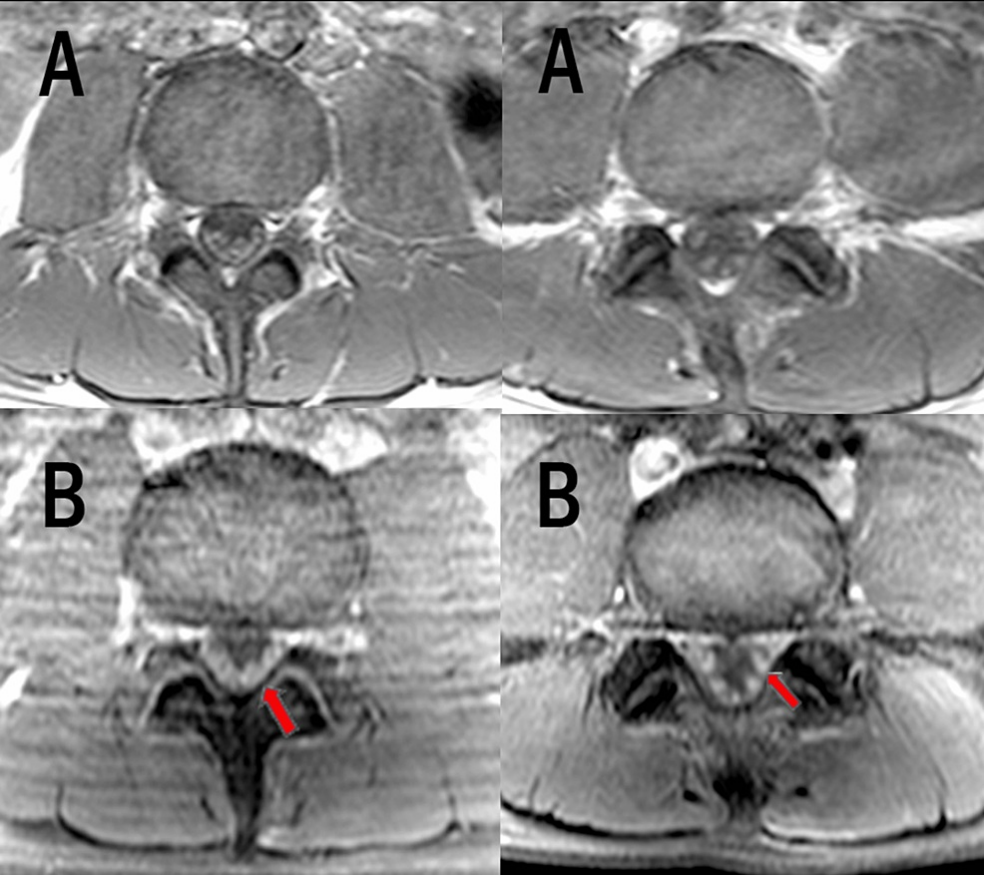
A and B show two levels of cauda equina. A (spine MRI axial T1 pre-contrast) and B (spine MRI axial T1 post-contrast) show contrast enhancement of cauda equina nerve roots (arrows). MRI: magnetic resonance imaging
